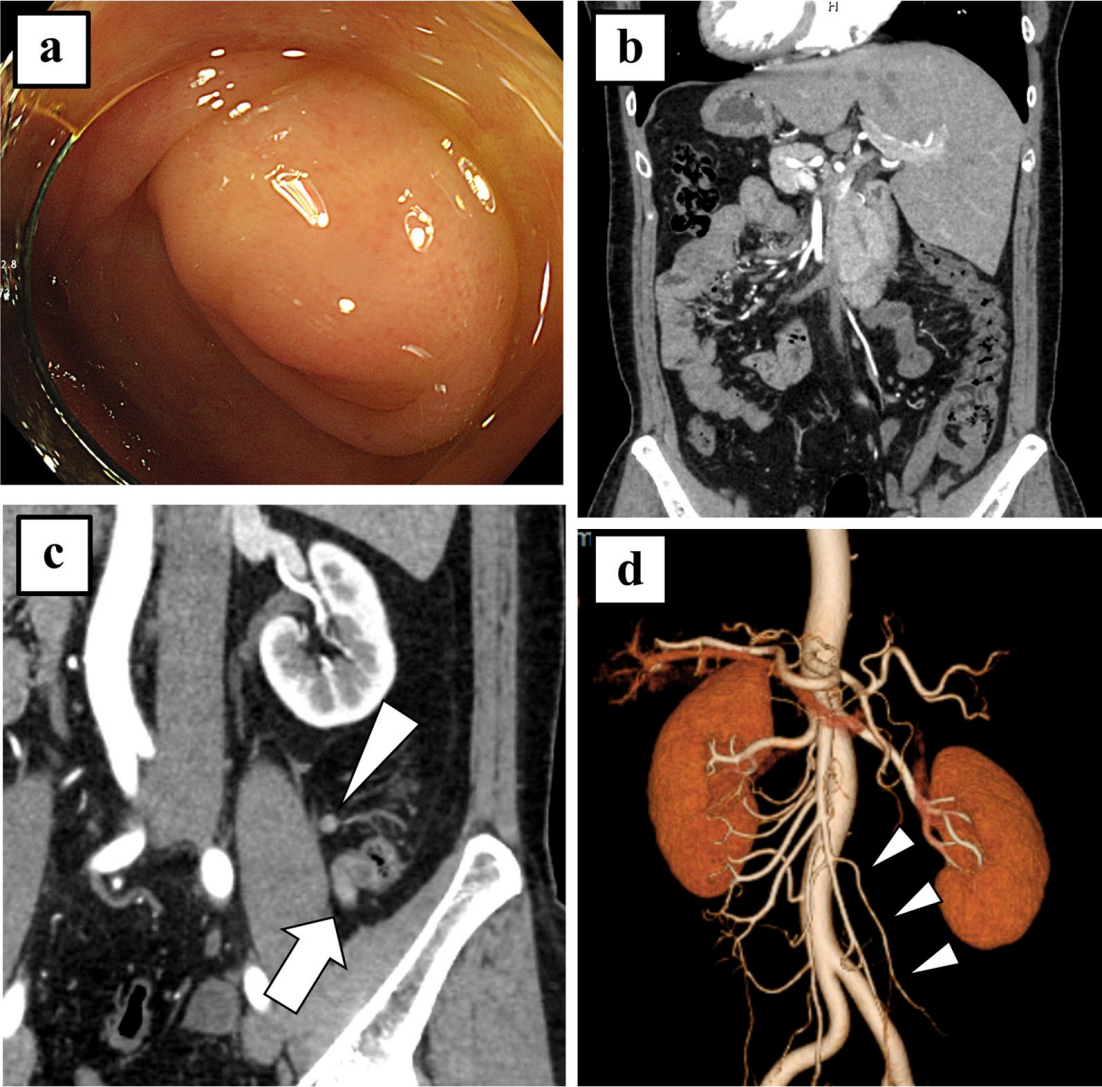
Preoperative examinations. a Colonoscopy showing a bulge at the appendiceal orifice. b Abdominal contrast-enhanced computed tomography (CT) showing complete “mirror-images” of the visceral organs; situs inversus totalis. c CT also showing appendiceal wall thickening, a cystic tumor with contrast effect (arrow), and an enlarged lymph node close to the tumor (arrowhead). d 3D-CT showing no vascular anomalies, except for completely inverted vessels. The arrow heads indicate the ileocolic artery
